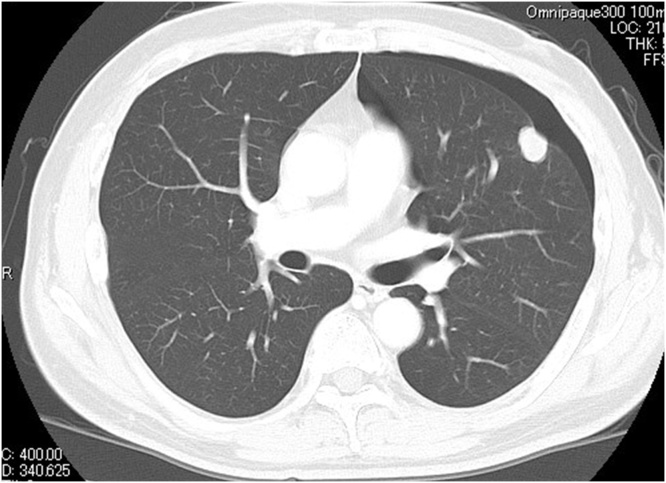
Chest CT showing left pneumothorax and a lesion measuring 18 mm in diameter in the inferior lingular segment of the lung.
